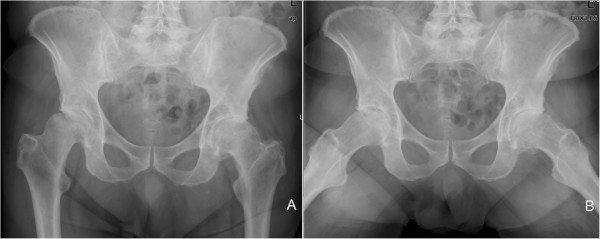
Antero-posterior (A) and frog-leg (B) pelvis radiographs showing sclerotic changes in both acetabulum, loss of articular joint distance, osteophytes and degenerative changes in both hips, which indicate severe degenerative coxarthrosis.
